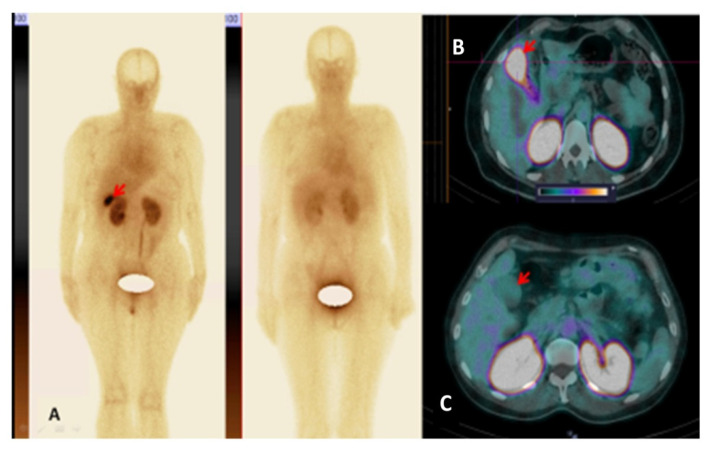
(A) Anterior images of female patient 5 (left) and female patient 4 (right) at 2 h after injection of [^99m^Tc]Tc-TG. High [^99m^Tc]Tc-TG uptake in the gallbladder in patient 5 is visualized (arrow). The upper setting of the scale window is 100 % of the maximum counts. (B) SPECT/CT image of patient 5 at 2 h after injection of [^99m^Tc]Tc-TG. High [^99m^Tc]Tc-TG uptake (SUVmax = 20.89) in the gallbladder in Patient 5 is visualized (arrow). (C) SPECT/CT image of patient 4 at 2 h after injection. There is no pathological uptake in the gallbladder of patient 4 (SUVmax = 2.87) (arrow). The upper setting of the scale window (100% of the maximum number).
